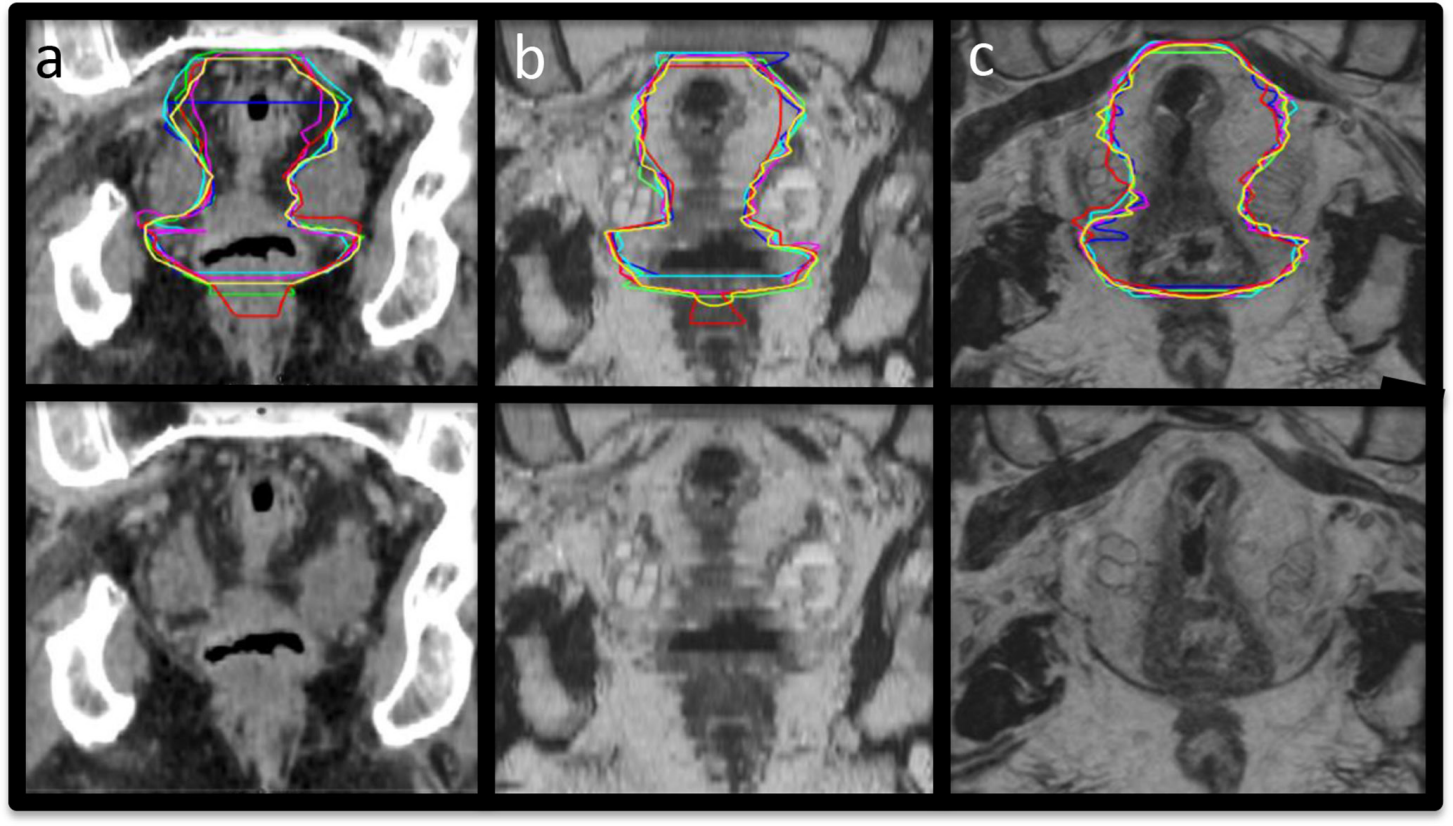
Coronal images of mesorectum with and without observer contours. (a) CT and (b) 2D-MRI and (c) 3D-MRI.
